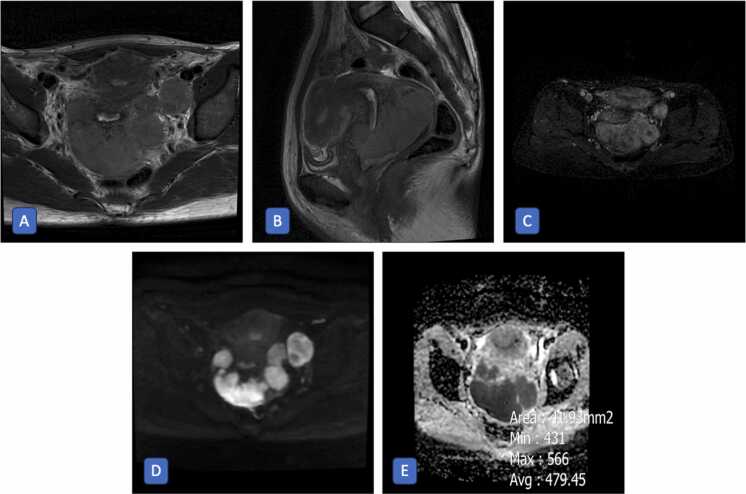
Axial T2-weighted (A), sagittal T2-weighted (B), subtraction T1-weighted post-contrast (C), axial diffusion-weighted (D), and apparent diffusion coefficient (ADC) (E) MR images of a 43-year-old woman with biopsy-proven cervical carcinoma. The images show a large cervical mass measuring approximately 97 mm in maximum diameter with parametrial and proximal vaginal extension A prominent left external iliac lymphadenopathy is also present, showing restricted diffusion on DWI. The primary cervical tumor demonstrates marked diffusion restriction with a corresponding low ADC value of 471 × 10⁻⁶ mm²/s, findings compatible with FIGO stage IIIC1 cervical carcinoma.
